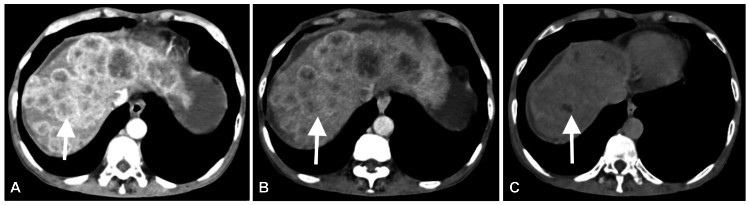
(A) There is one suspicious nodule in the right lobe of the liver (white arrow), showing peripheral enhancement on the arterial phase of the CT abdomen. (B) The suspicious nodule appears isoattenuating on the venous phase of the CT abdomen. (C) The suspicious nodule shows washout and appears hypodense in the delayed phase. These patterns of contrast enhancement on the arterial, venous, and delayed phases of the contrast CT abdomen suggest hepatocellular carcinoma.
